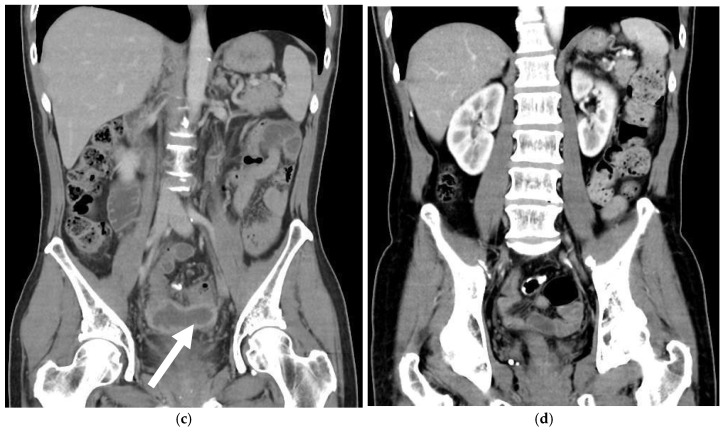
Serial contrast-enhanced abdominal CT images demonstrating the clinical course of an intra-abdominal abscess following sigmoid neovaginoplasty. (a) Axial contrast-enhanced CT image obtained at initial presentation reveals a large intra-abdominal abscess occupying a significant portion of the pelvic cavity. The abscess is well circumscribed with rim enhancement, indicating mature, encapsulated fluid collection. The white arrows highlight the abscess cavity abutting the neovaginal wall, suggestive of potential neovaginal origin or involvement. (b) Axial CT image obtained six months after appropriate surgical and medical management shows complete resolution of the abscess. The neovagina appears patent, without evidence of perforation, surrounding inflammation, or fluid re-accumulation. (c) Sagittal view of the abdomen on contrast-enhanced CT at the time of diagnosis demonstrates the same encapsulated intra-abdominal abscess located in the rectovaginal space. The fluid collection is again well circumscribed with an enhancing capsule, as indicated by the white arrow, compressing adjacent structures. (d) Follow-up sagittal CT image six months post-treatment confirms resolution of the abscess. The neovagina remains intact and clearly delineated, with no signs of perforation or residual infection, indicating successful treatment and structural preservation.
